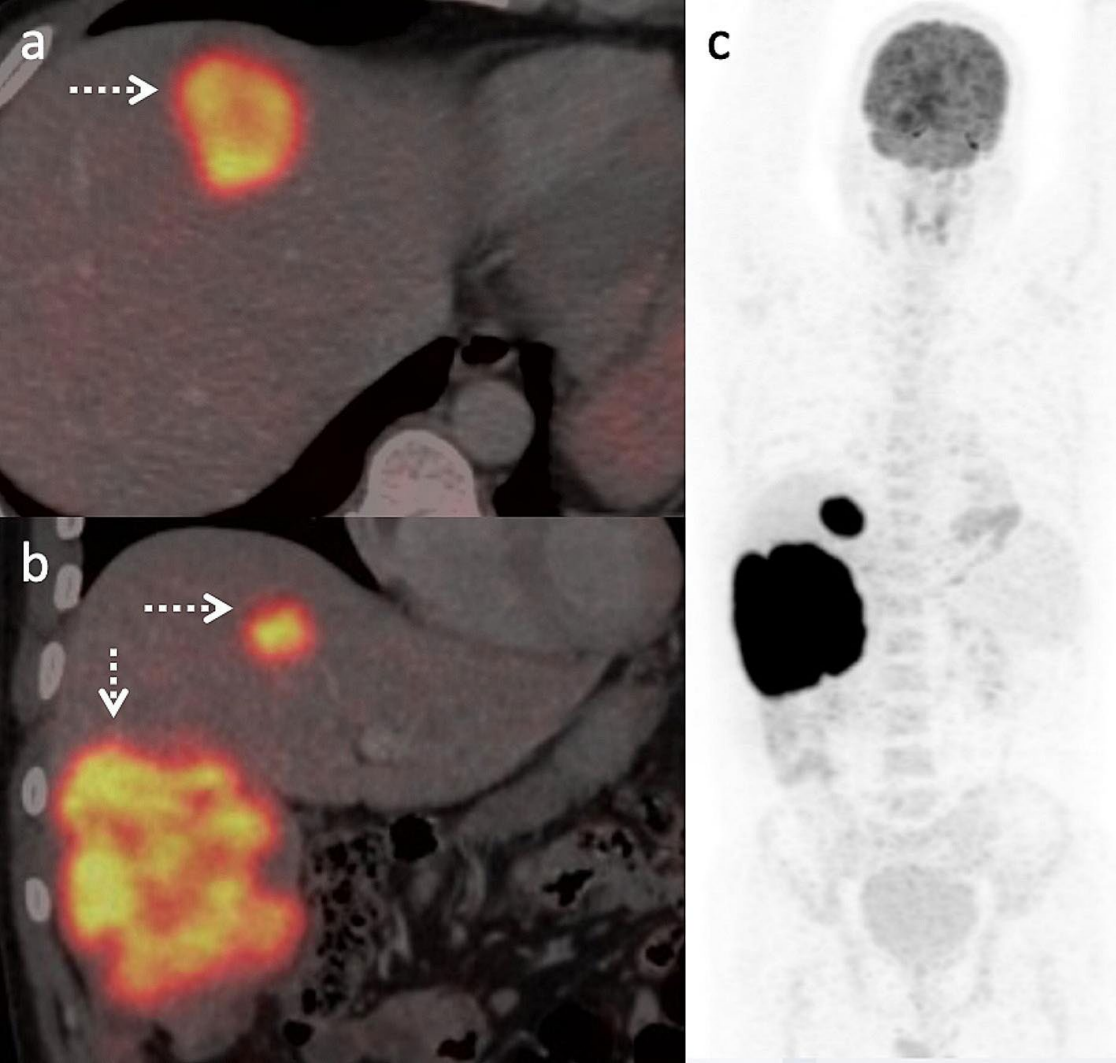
Fused axial (a) and coronal (b) along with maximum intensity projection (c) images of F-18 FDG PET/CT showed intense metabolism in these hepatic lesions (arrows)
